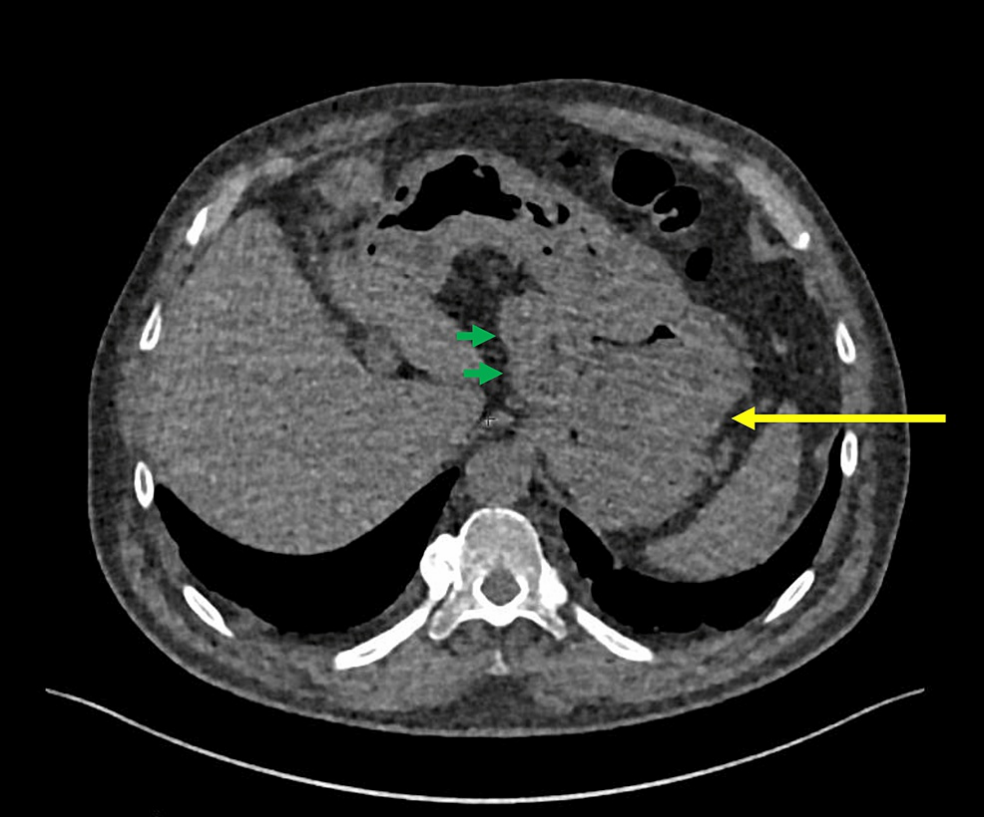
Lower section of unenhanced chest CT Retrospectively, the gastric metastases (yellow arrows) could have been only suspected in the lower sections of the last follow-up unenhanced chest CT, which was performed one month before the MRI. However, the CT was incapable of definitively diagnosing the condition. Moreover, the two necrotic adenopathies (green arrows) were clearly visible against the lesser curvature of the stomach.
